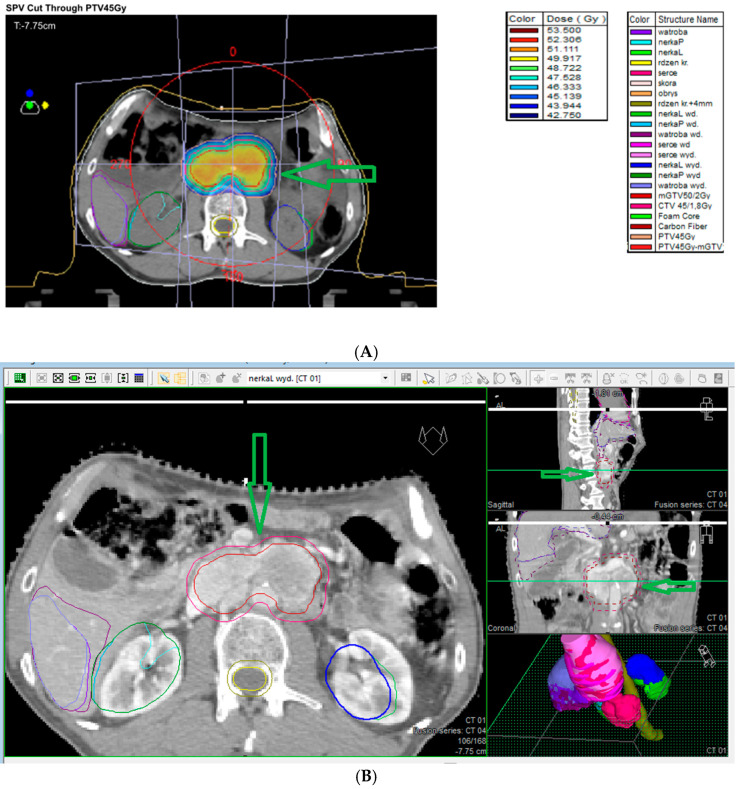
(A) Conventional radiotherapy for retroperitoneal paraganglioma in a 52-year-old white male patient. Dose distribution shown on the CT scan; and (B) conventional radiotherapy for retroperitoneal paraganglioma in a 52-year-old white male patient. CT for treatment planning.
